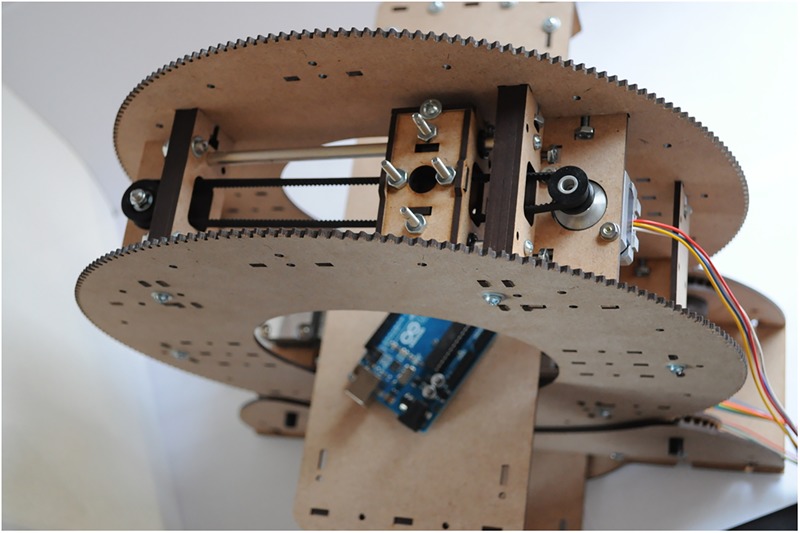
Open-source CT scanner (Photo by Peter Jansen, image licensed under the Creative Commons BY-SA license).
